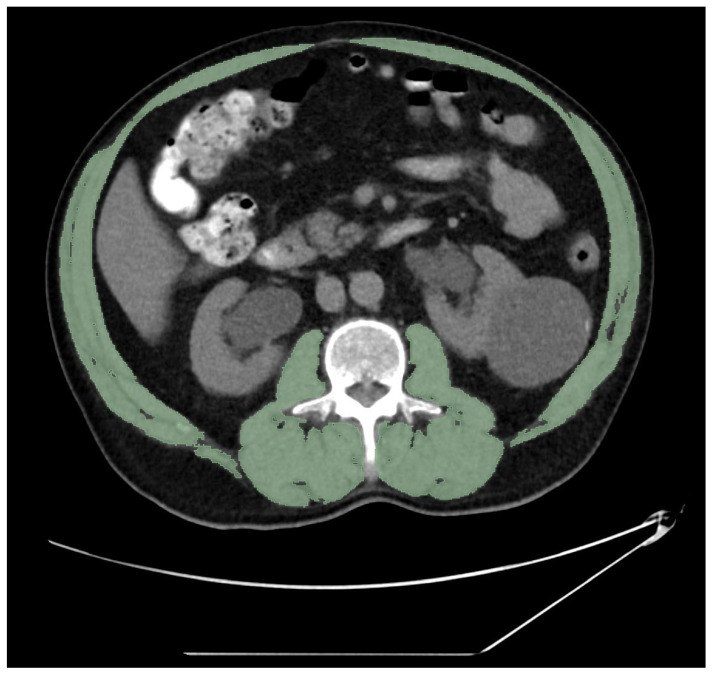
Representative axial CT image at the L3 level showing skeletal muscle area segmentation. The highlighted region represents the segmented skeletal muscle compartment used for SMI calculation. Segmentation was performed using a −30 to +150 HU threshold, followed by manual correction when necessary.
